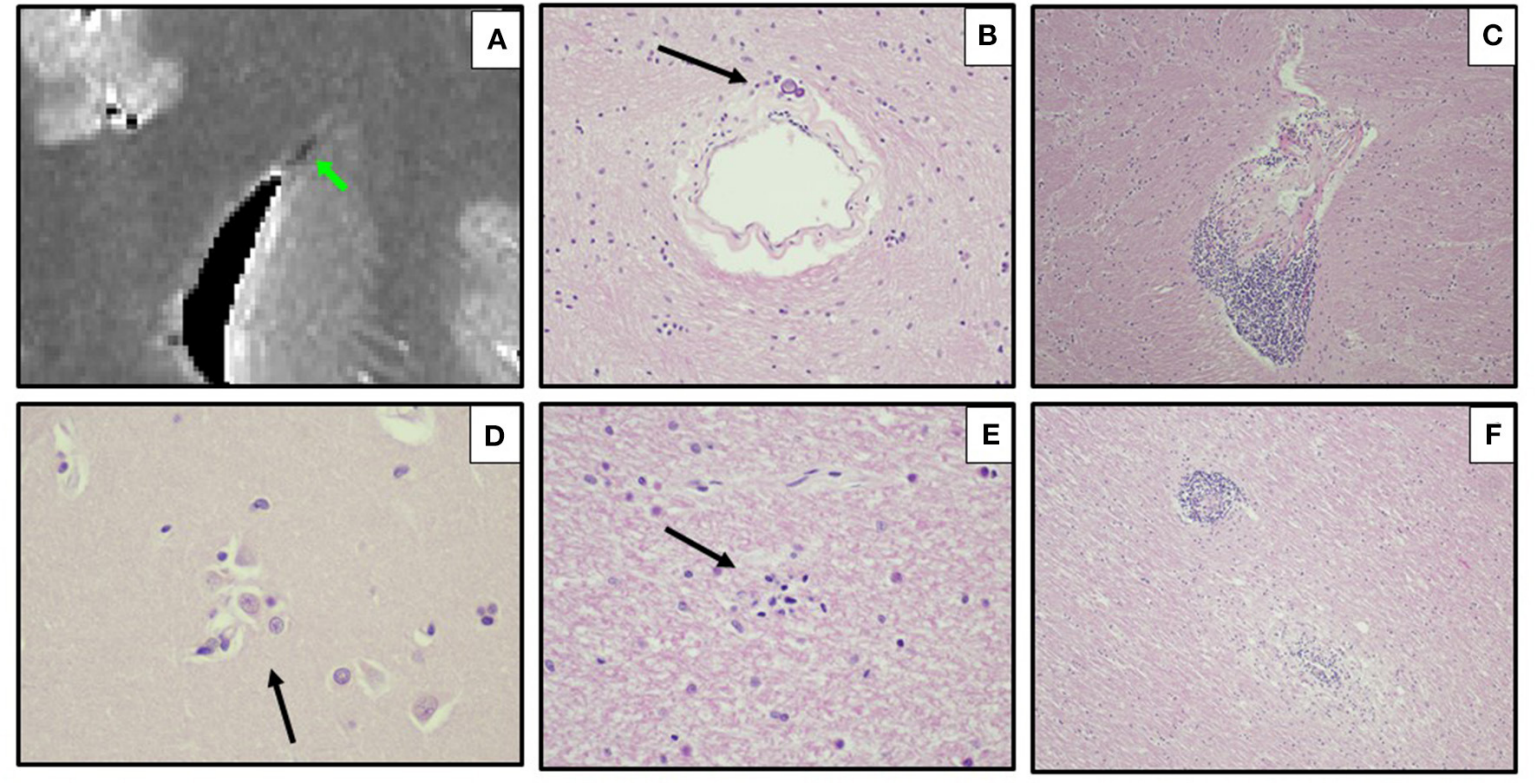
Ex vivo* MRI and autopsy neuropathology in Case 8. (A)
